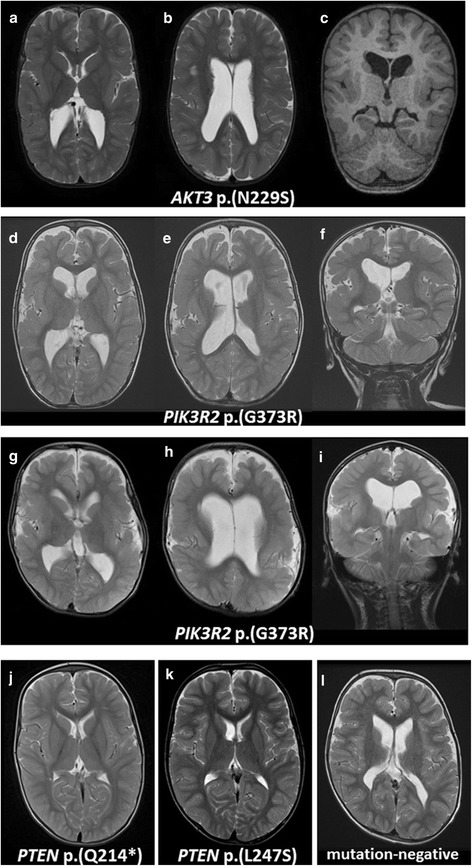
MRI findings in PI3K-AKT-mTOR pathway-associated megalencephaly. T2-weighted axial images (a, b, d, e, g, h, j-l), T1-weighted coronal image (c), T2-weighted images (f, i) of patients (a-c, Patient 1 with an *AKT3* mutation [p.(N229S)] at 2 years of age; d-f, Patient 2 with a *PIK3R2* mutation [p.(G373R)] at 2 years; g-i, Patient 3 with a *PIK3R2* mutation [p.(G373R)] at 6 years; j, Patient 4 with a *PTEN* mutation [p.(Q214*)] at 2 years; k, Patient 5 with a *PTEN* mutation [p.(L247S)] at 1 year and 9 months; l, Patient 8 without mutation at 4 years. In Patient 1–3, ventriculomegaly (b, e, h), bilateral polymicrogyria that appears to be most severe in the perisylvian regions but is also present in other regions (a, c, d, f, g, i), and an abnormally high intensity signal from white matter (b, e, h) were observed. The patient with *PTEN* mutations showed no abnormalities (j, k). Patient 8, without mutation, showed only mild ventriculomegaly (l)
